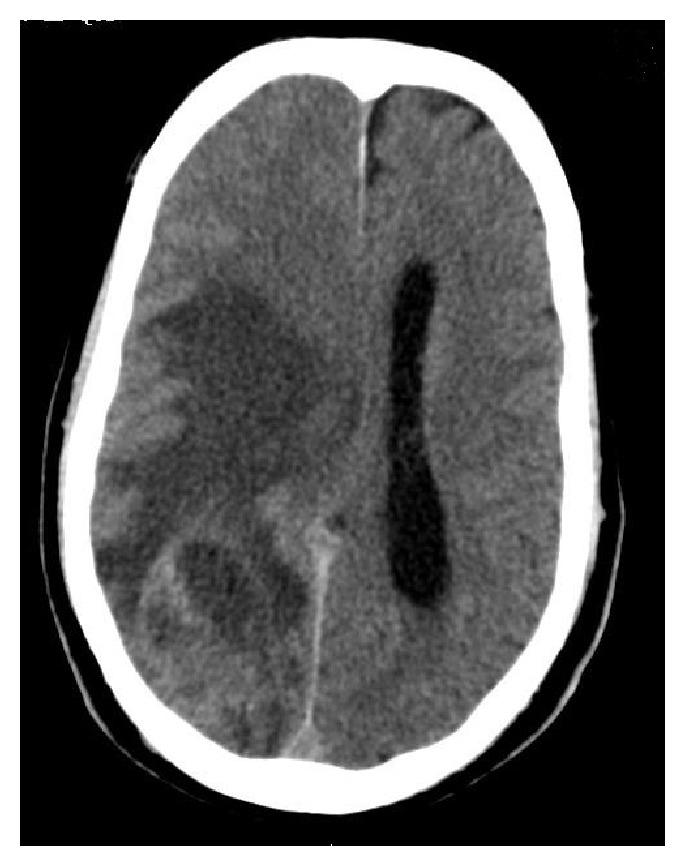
Head CT: a heterogeneous lesions, mostly marginal contrast enhancement (50 × 40 × 40 mm) with large mass effect, a vast zone of grade III edema, covering the structures of the right hemisphere and displacing the middle cerebral structures towards the left (11 mm) in the parietooccipital part of the right hemisphere of the brain. A similar minor (13 mm) change was present in the left frontal lobe with a small area of swelling and in the subtentorial area, in the left cerebellar hemisphere (16 mm).
